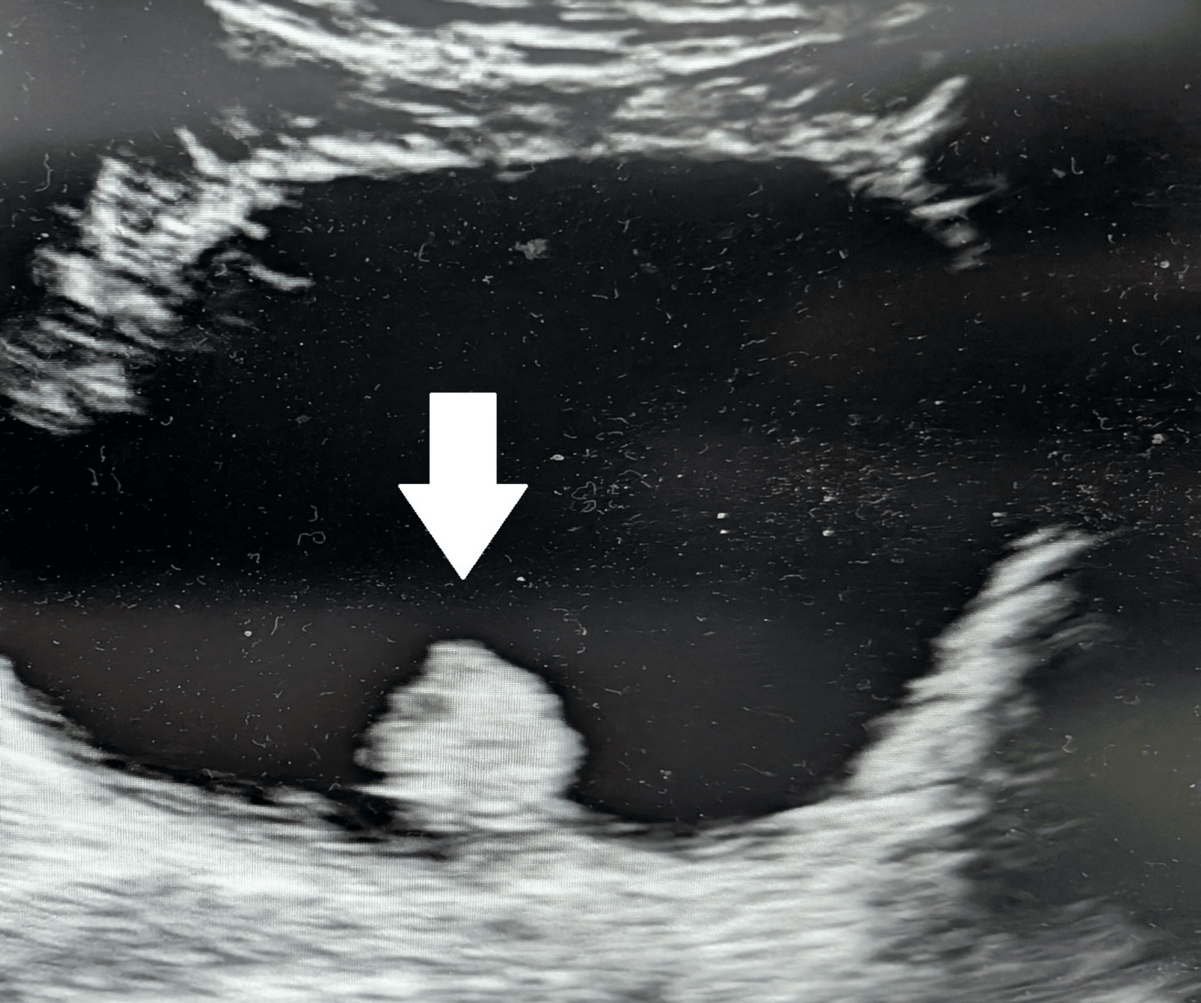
Ultrasound reveals a solitary 3-cm intravesical mass arising from the posterior wall in the trigonal region (Case 1) Bladder ultrasound demonstrates a polypoid, echogenic intraluminal lesion projecting from the posterior wall in the trigonal region (arrow). The lesion outlines smoothly toward the bladder lumen without acoustic shadowing, in keeping with an intravesical mass (~3 cm) later confirmed endoscopically.
